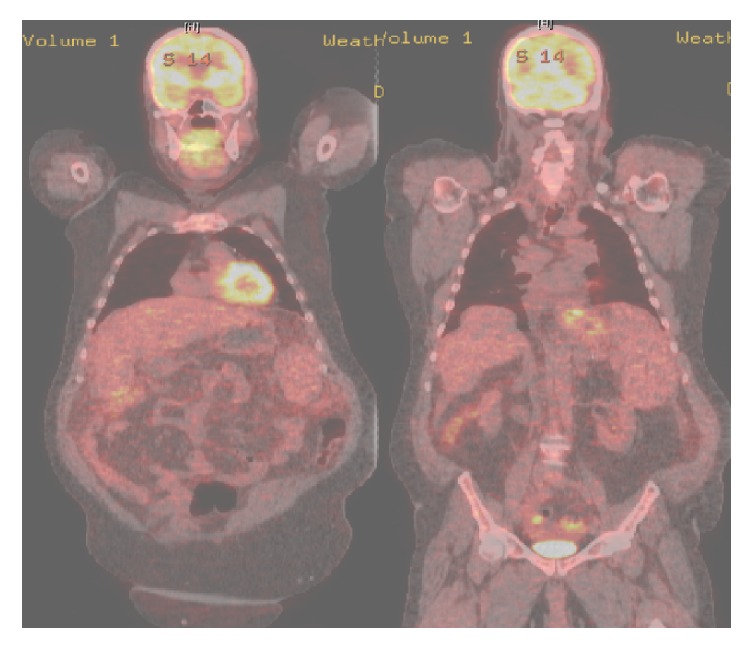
PET CT scan showing the absence of focal FDG uptake, in addition to stigmata of liver cirrhosis.
